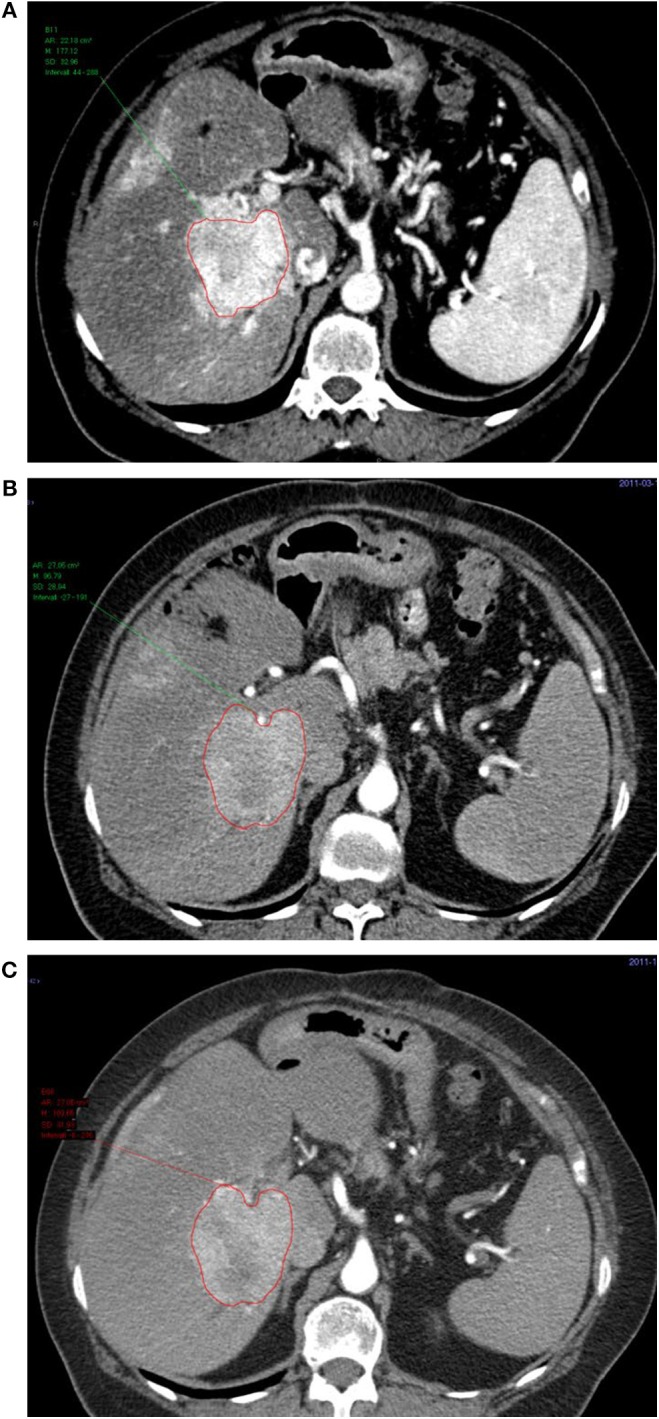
Transversal contrast-enhanced CT images in the arterial phase of a liver metastasis at baseline (A), before the 3rd cycle of treatment (B), and at follow-up (C).
